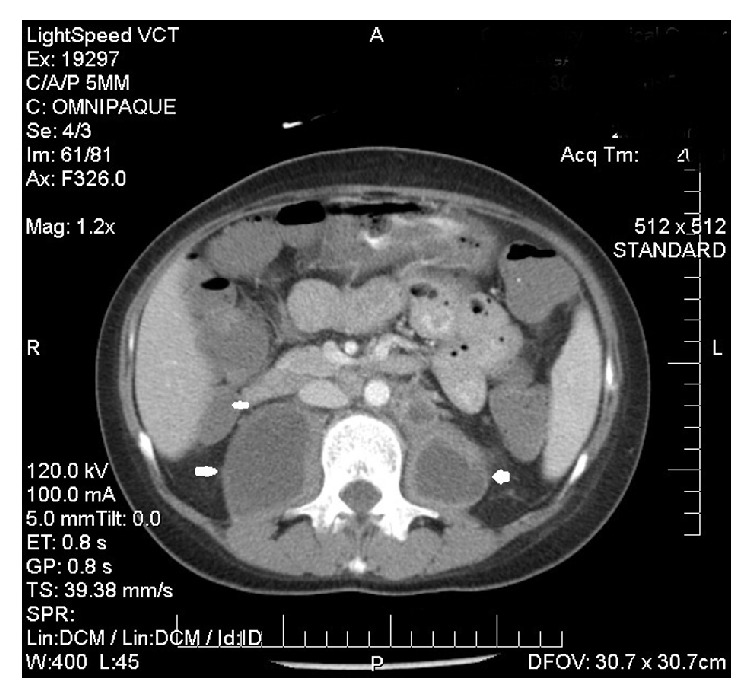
Abdominal CT scan demonstrating large hypodense iliopsoas fluid collections (white arrows).
